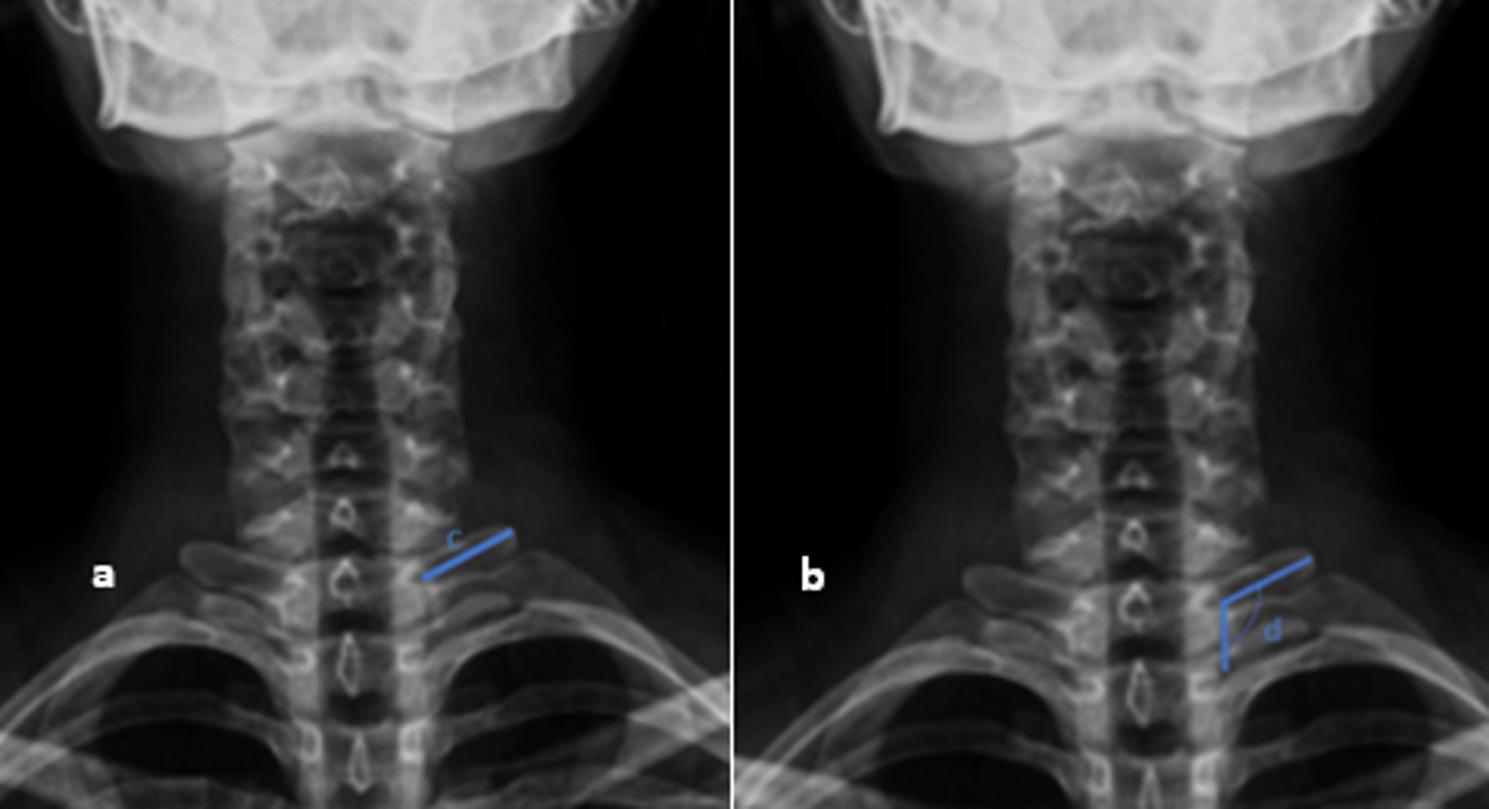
Measurement of T1 transverse process length and angle with vertebral body on cervical AP X-ray (a) The length of the T1 transverse process was defined as the distance between the lateral margin of the T1 vertebral body and the most lateral tip of the T1 transverse process. (b) The angle between the T1 transverse process and the T1 vertebral body was measured as the angle formed between the longitudinal axis of the T1 vertebral body and its lateral margin
